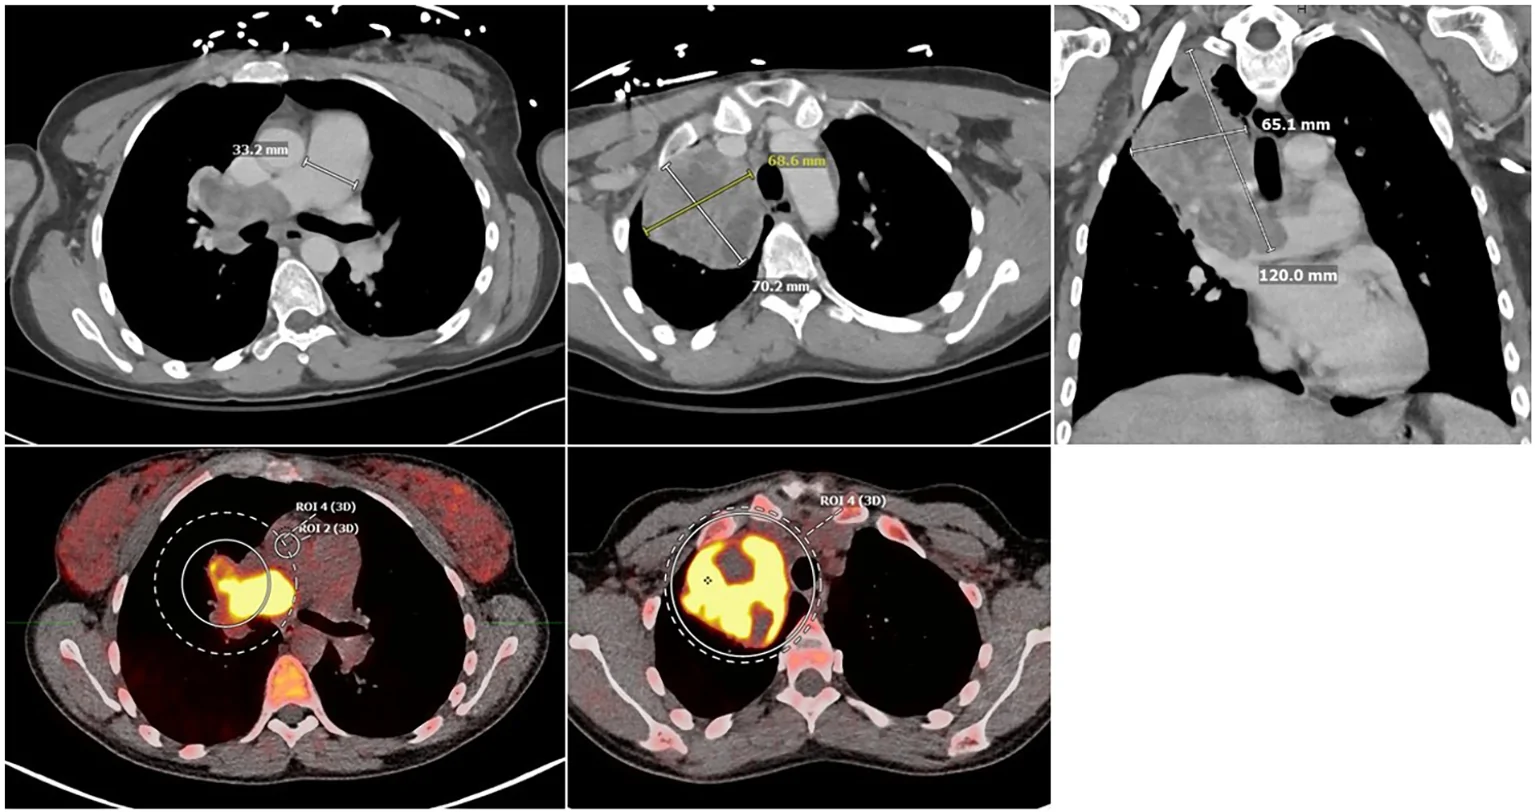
CT and PET-CT performed before pembrolizumab treatment, demonstrating maximal dimensions of the lesion and pulmonary artery involvement.
